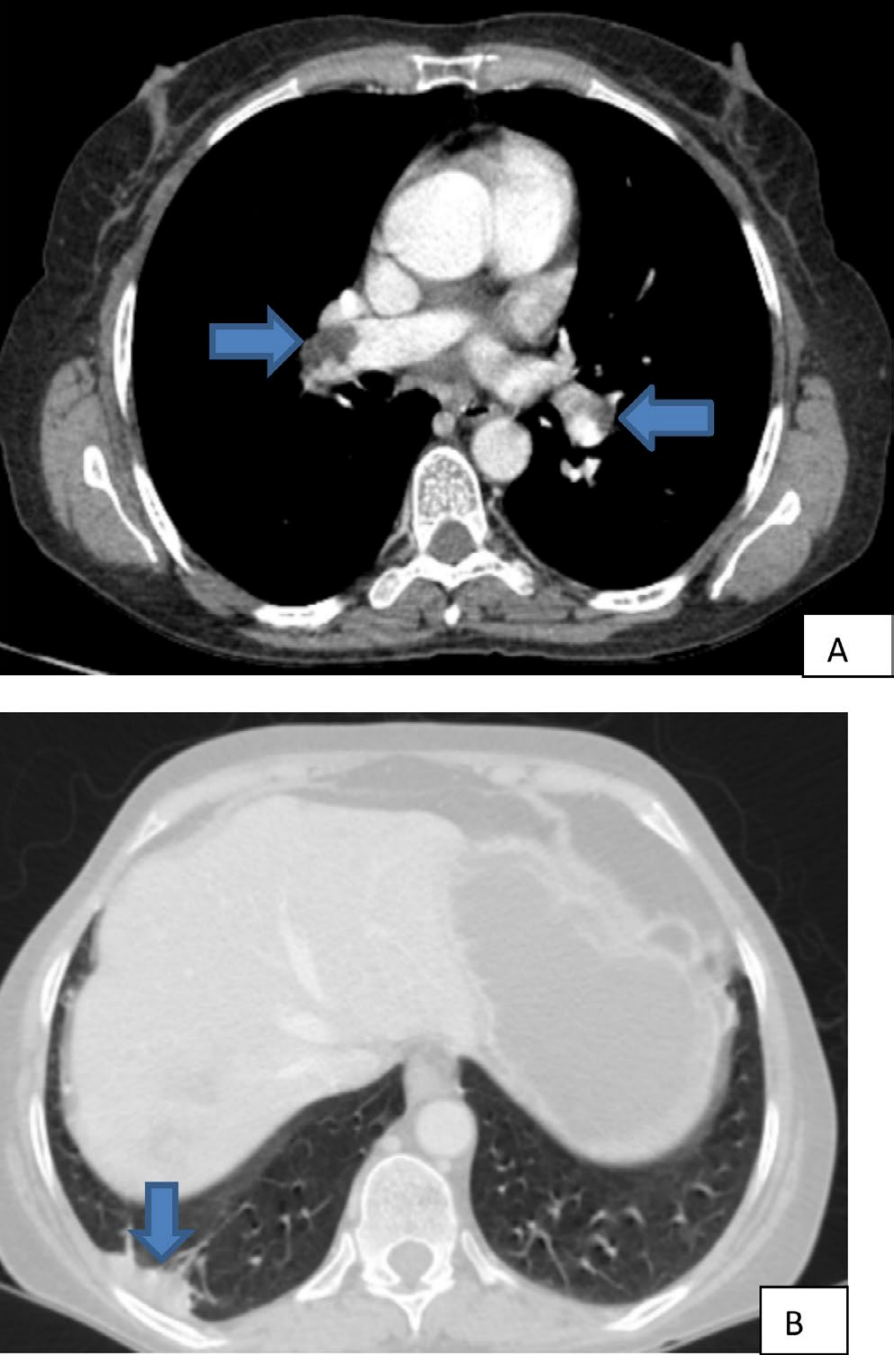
(A, B) Contrast‐enhanced chest computed tomography: (A) filling defect noted within the right main pulmonary artery and within the left descending pulmonary artery; (B) peripheral based air space opacity noted in the right basal segment of the lower lobe.
